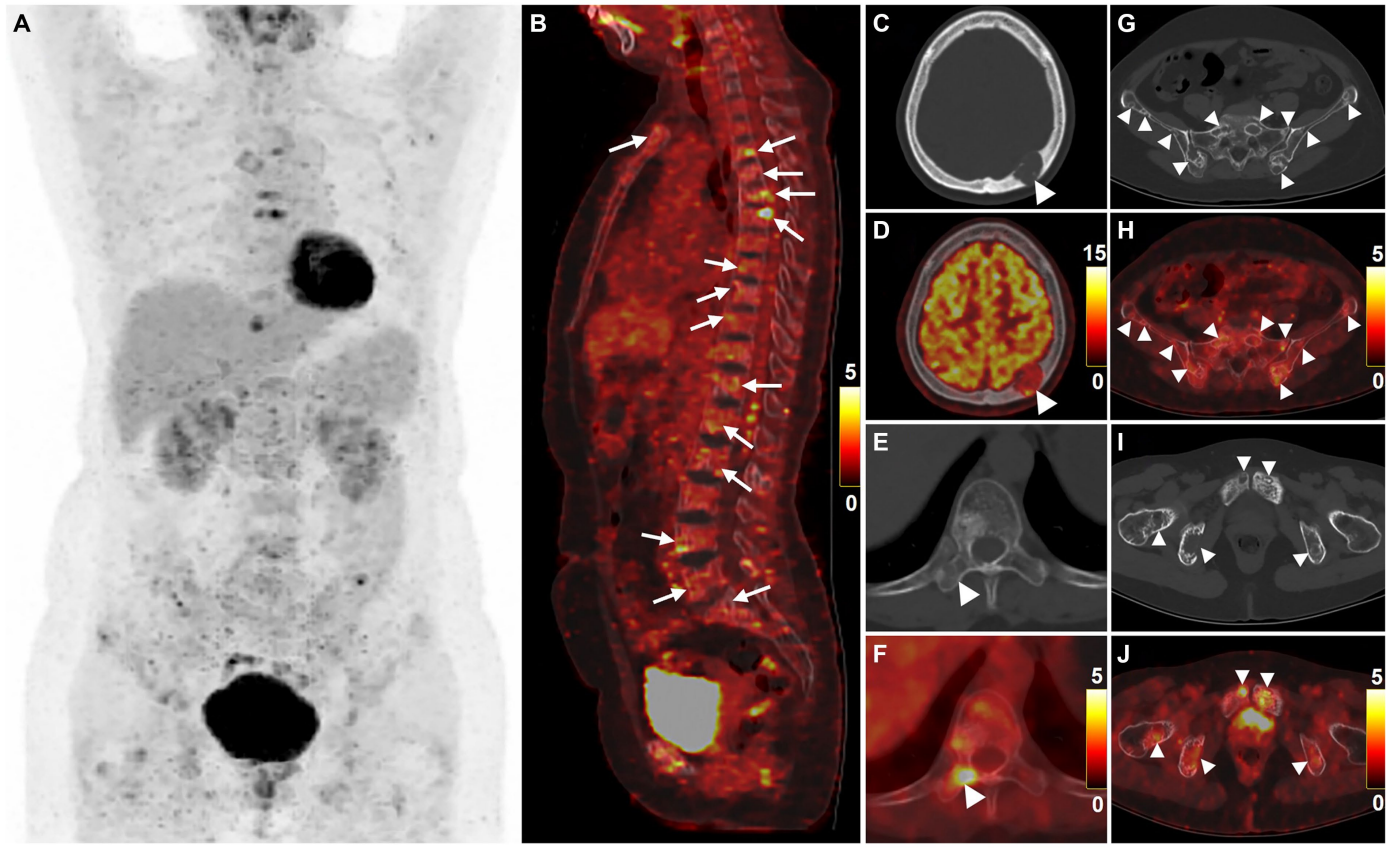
^18^F-FDG PET/CT images of multicentric angiosarcomas of bone. The anteroposterior 3-dimensional maximum intensity projection image (MIP) revealed multifocal FDG-avid lesions in the sternum, bilateral clavicles, bilateral scapulae, multiple ribs, and pelvic bones (A). Sagittal images of the spine revealed the presence of multiple bone-destroying lesions (long arrows), with the highest FDG uptake detected in the T6 vertebra (B, SUVmax = 7.6). Heterogeneous FDG uptake was observed in multiple bone lesions (arrowheads), including intracranial (C,D, SUVmax = 11.3), right transverse process of the T10 vertebra (E,F, SUVmax = 5.8), ilium (G,H, SUVmax = 3.3), and pubis (I,J, SUVmax = 4.7).
